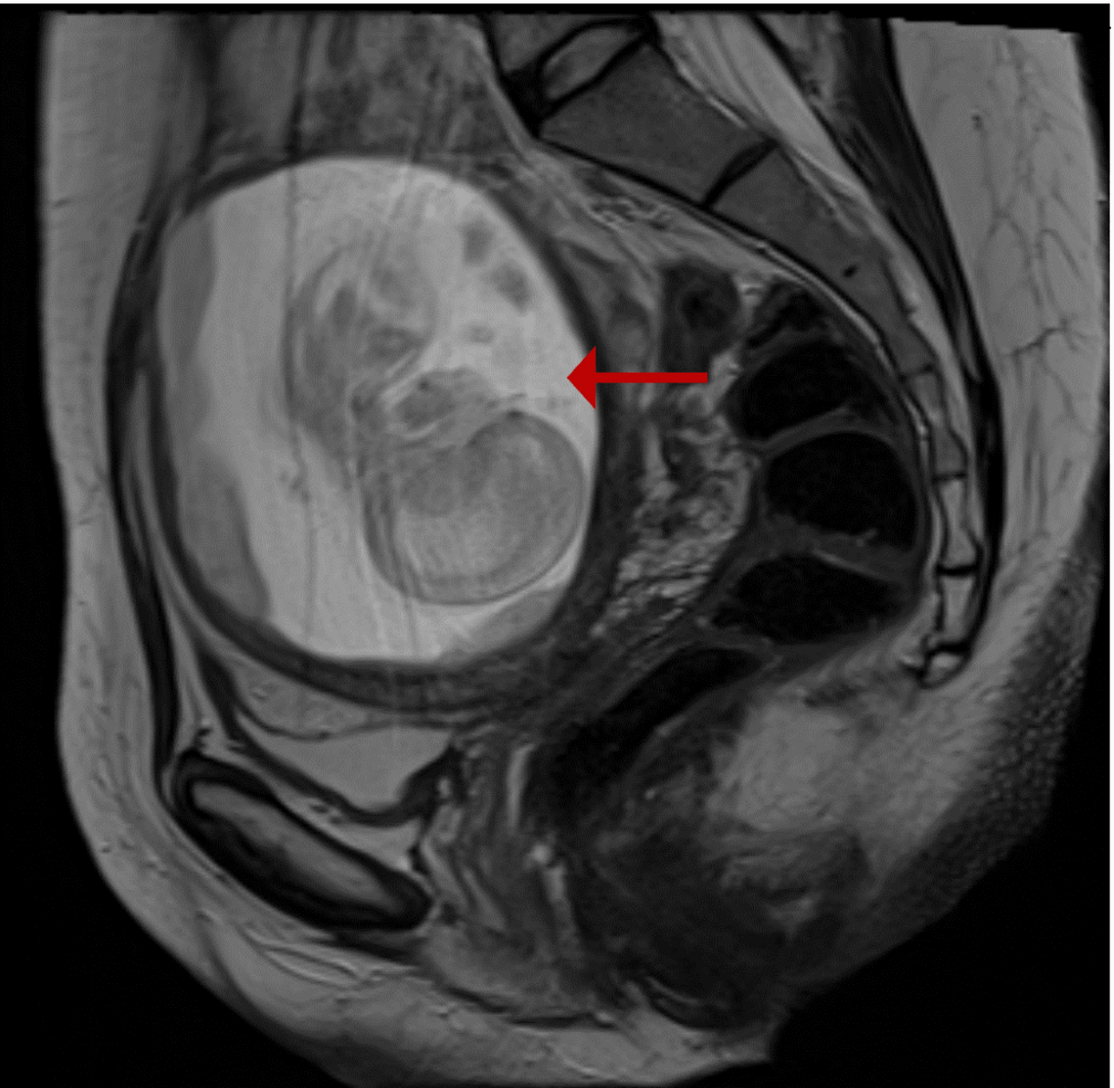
Sagittal section of MRI pelvis showing rudimentary horn containing fetus (red arrow).
